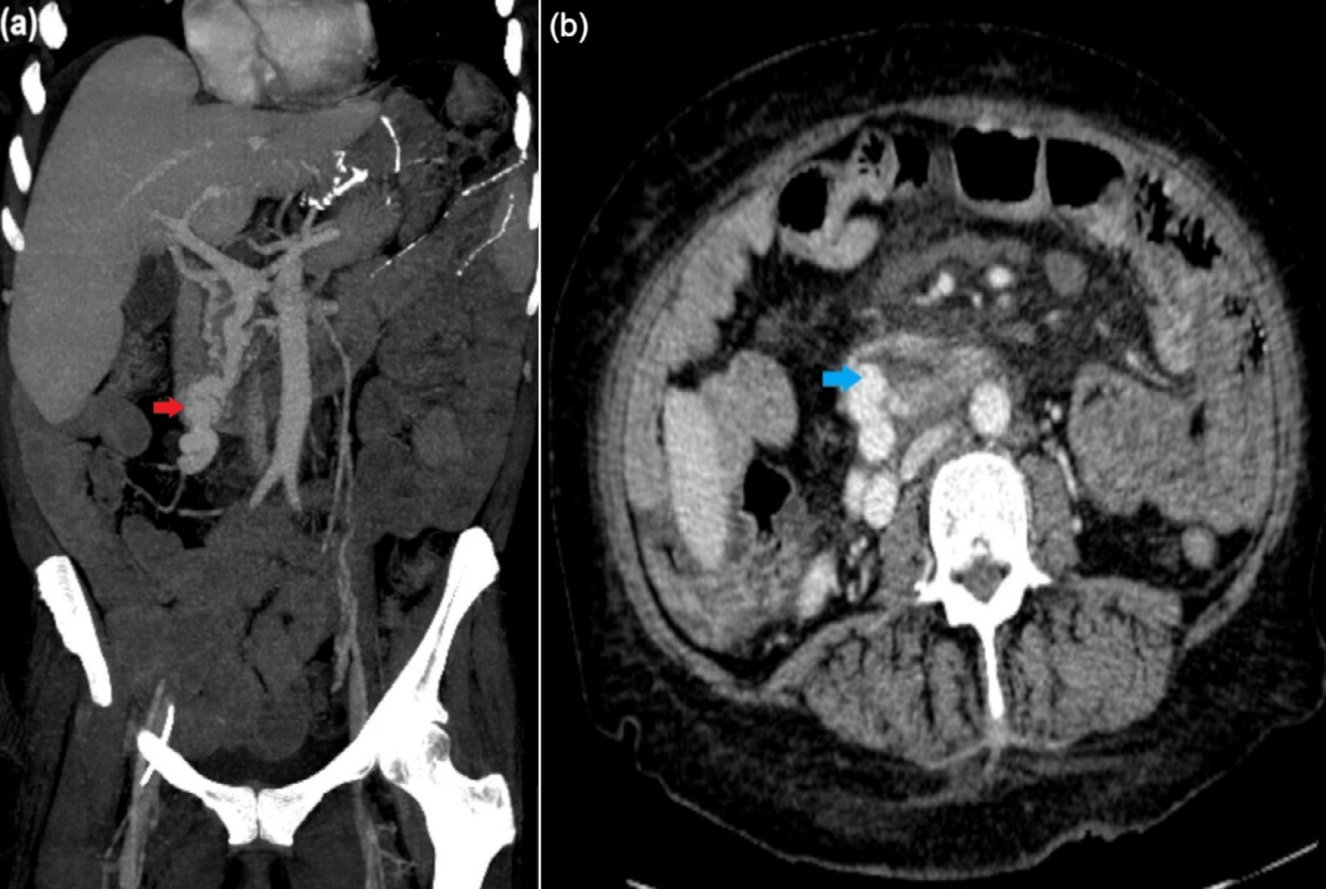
CT images prior to percutaneous transhepatic embolization and TIPS. (A) Coronal post intravenous (IV) contrast CT image through abdomen and pelvis demonstrating a large duodenal varix (red arrow) with periduodenal edema. (B) Axial post IV contrast CT image demonstrating duodenal varix protruding into duodenal lumen (blue arrow).
